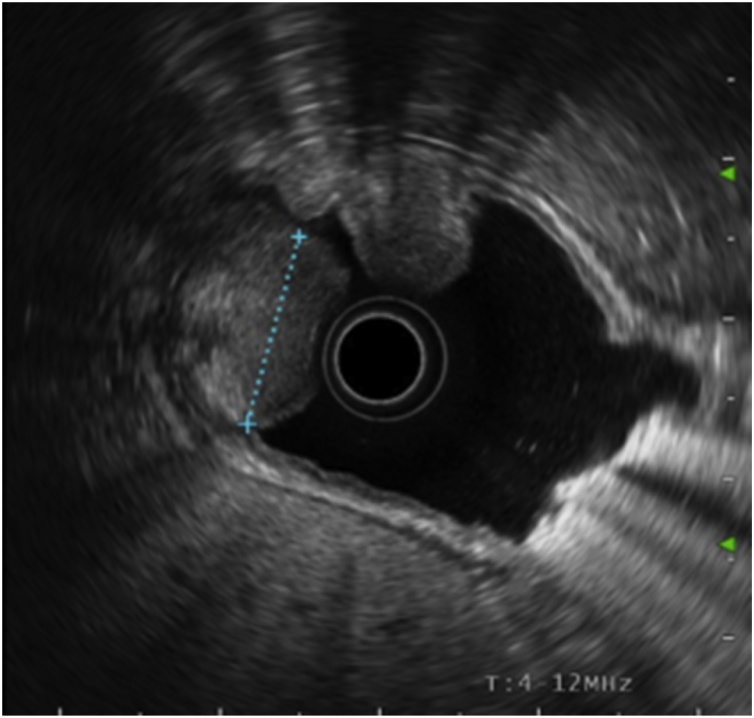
Endoscopy ultrasound: huge fat-containing mass lesion well-defined, homogeneously hyperechoic with a maximun size of 27 × 17 mm.
